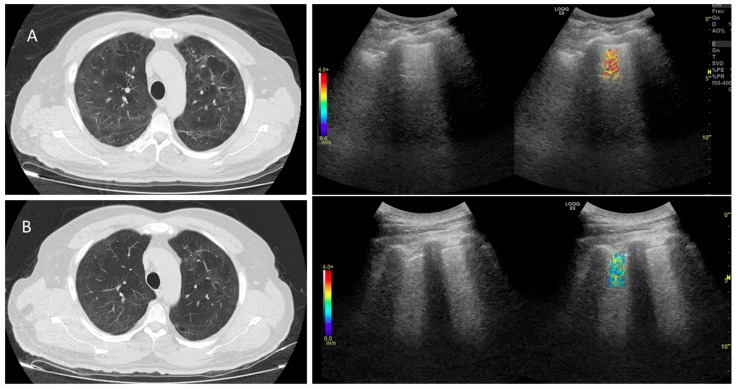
Ultrasonography on B mode, without/with elastography, and Computed Tomography images of a 62 y.o. male after 112 and 209 days since hospital discharge. The shear wave velocity measured on the left lung by SWE2D was 3.58 m/s in the first evaluation (A), and 2.20 m/s in the second evaluation (B). The decrease in the stiffness (color red in (A)) was consistent with a parallel decrease in the “fibrosis like” signs observed by Computed Tomography.
